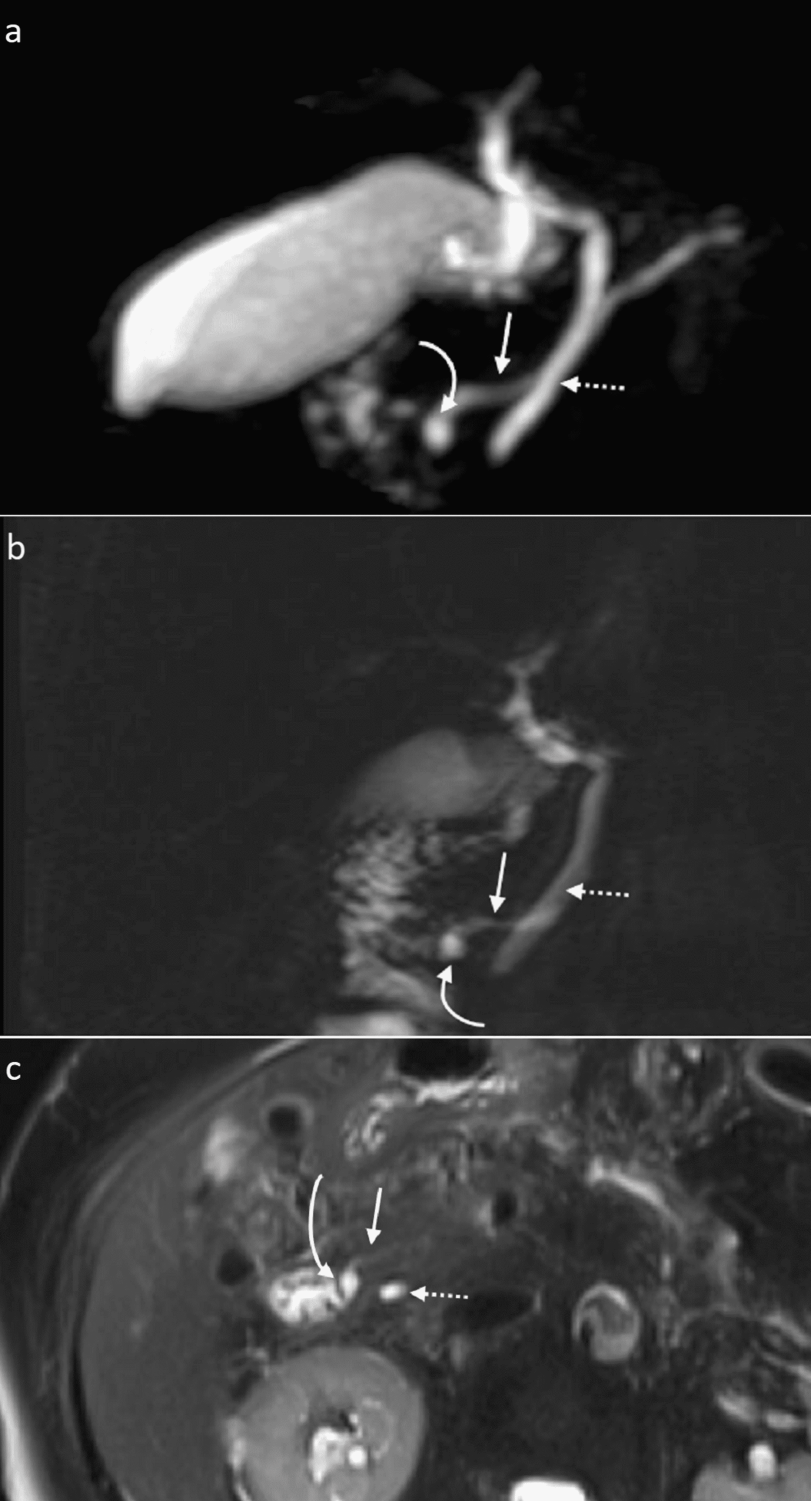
MRCP thick slap (a, b) and T2-weighted fat-saturated axial view (c) show focal dilatation indicative of Santorinicele (curved arrow) at the terminal end of the dorsal duct (straight arrow), which drains into the minor papilla. The common bile duct (dotted arrow) is seen draining separately into the major papilla. MRCP: magnetic resonance cholangiopancreatography
